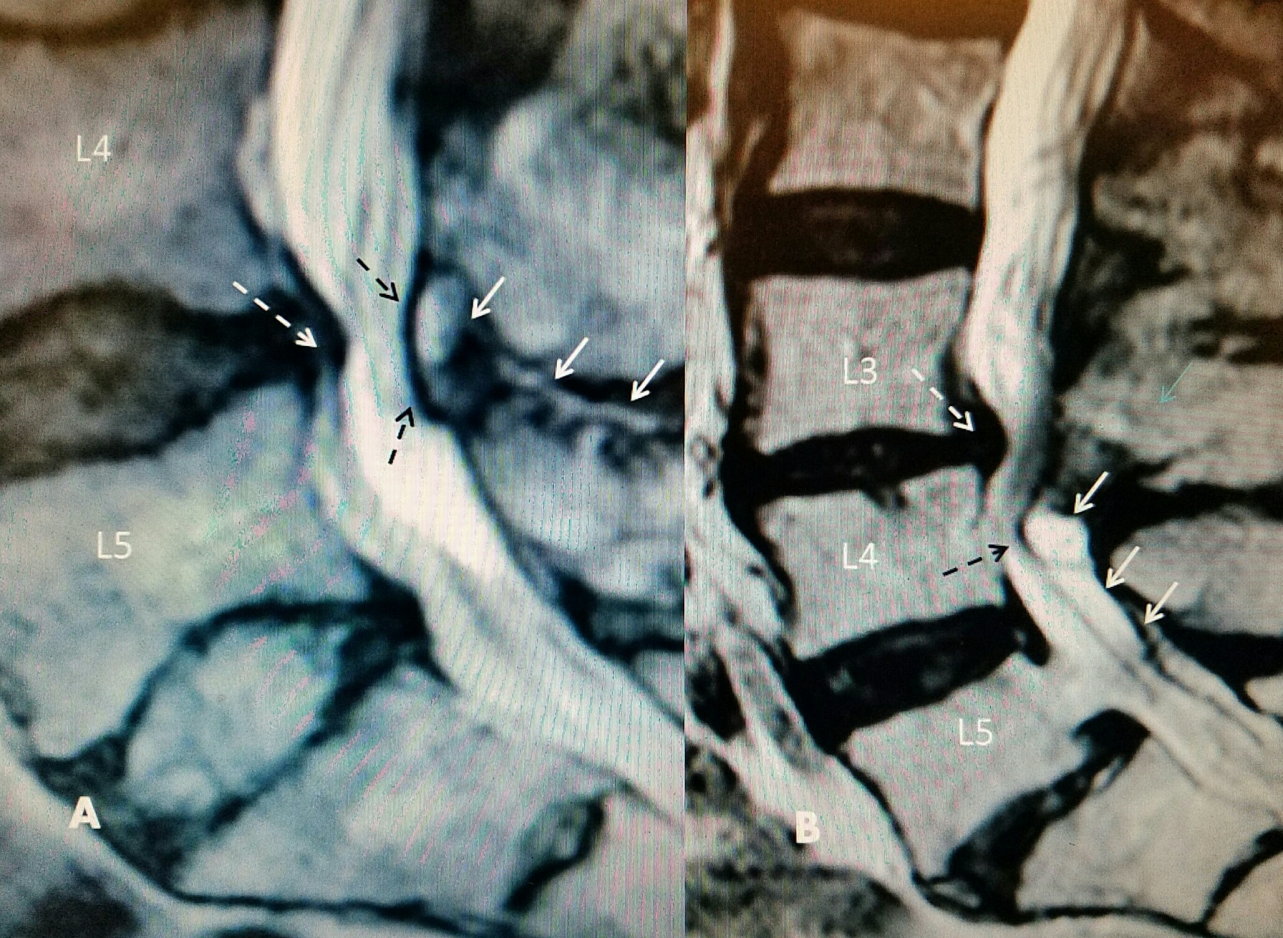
T2 sagittal magnetic resonance imaging (MRI): L4-5 disc degeneration with posterior dorsal cyst and interspinous hyperintense fluid A: Interspinous hyperintense T2 fluid signal at L4-5 (solid white arrows) leading into posterior epidural cyst causing lumbar stenosis (dashed black arrows). There is associated disc desiccation and posterior annular bulging (dashed white arrow). B: L3-4 grade 1 spondylolisthesis and annular bulge (dashed white arrow) with narrowed L3-4 disc space with large posterior cyst (uppermost solid white arrow) with T2 hyperintense signal inferior to hypertrophied ligamentum flavum (middle and lower solid white arrows). Cauda equina compression at L4 level (dashed black arrow). Separate grade 1 spondylolisthesis at L5-S1.
